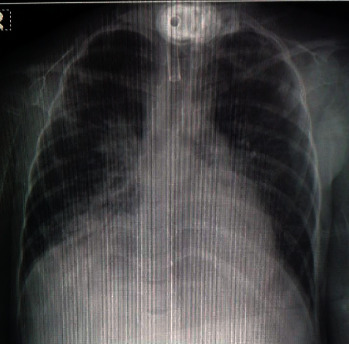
Chest X-ray prior to COVID-19: clear lung fields bilaterally with minimal veiling of the right middle lobe, tracheostomy in situ.
